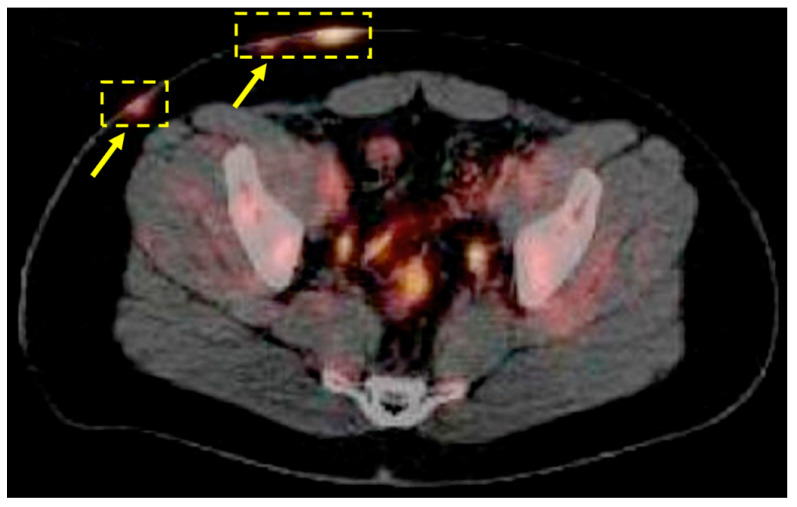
Positron emission tomography imaging of hidradenitis suppurativa (HS). 18-Fluorodeoxyglucose (FDG) was administered to visualize Hodgkin’s lymphoma in this hidradenitis suppurativa (HS) patient. There was substantial FDG uptake in the areas with HS lesions, inside of the dashed boxes, which resulted in the first documented case of positron emission tomography (PET) imaging of HS. Reproduced from Simpson et al., 2011 [32].
